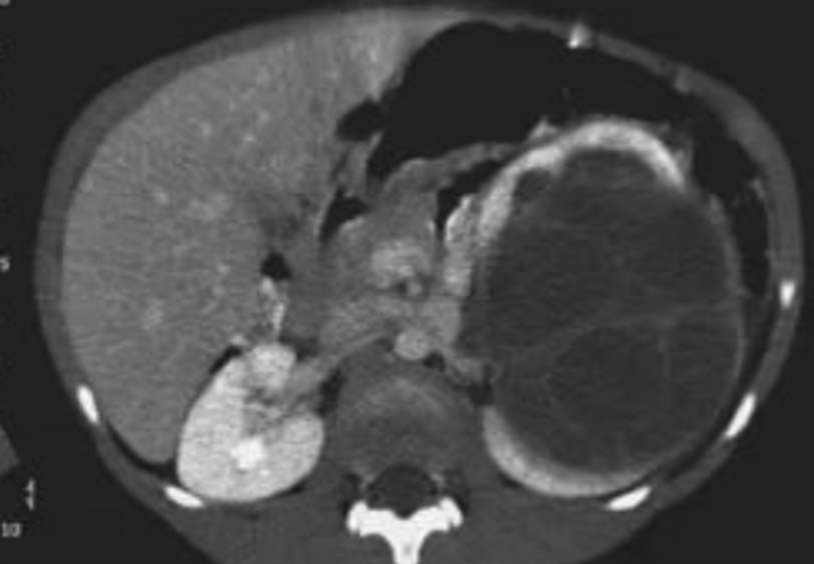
Computed tomography scan, a large multilocular cystic mass arising from the lower pole of the left kidney.
